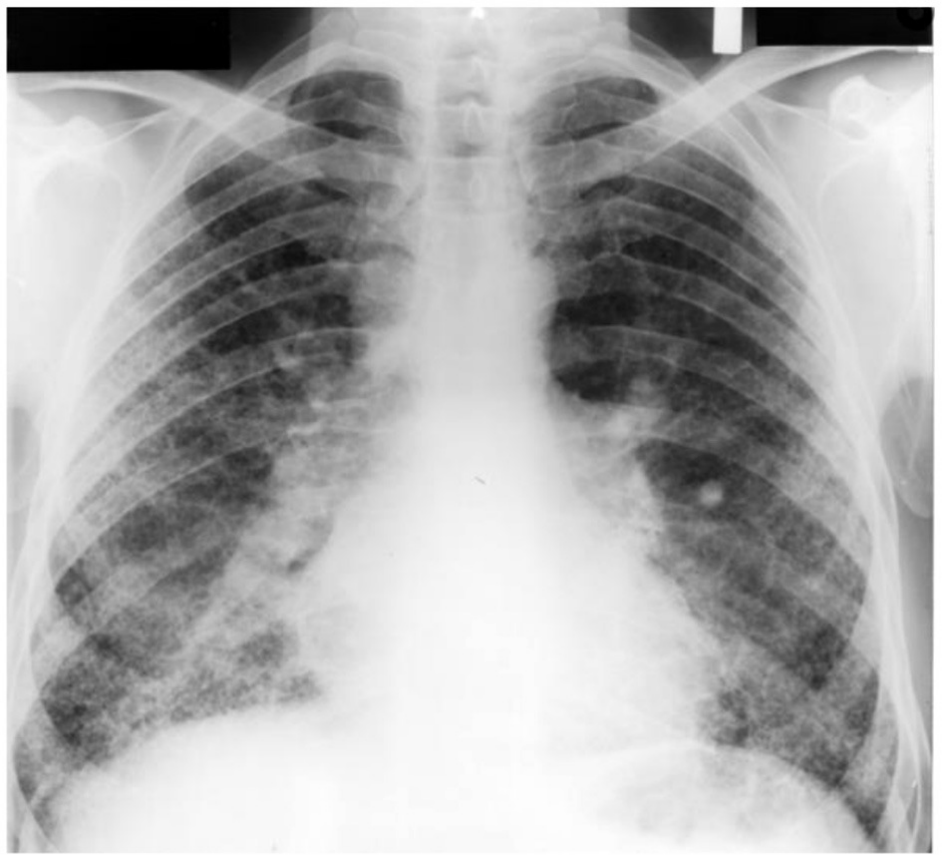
A chest radiograph of inflammatory and fibrotic injury following asbestos exposure. This is characterized by bilateral, small irregular opacities involving the lower lobes of the lungs. The dimensions of asbestos preclude efficient clearance (“frustrated phagocytosis”). Subsequently, in contrast to particles, the associated inflammation and fibrosis is in the lower lung fields where delivery is greater relative to the upper lung fields.
